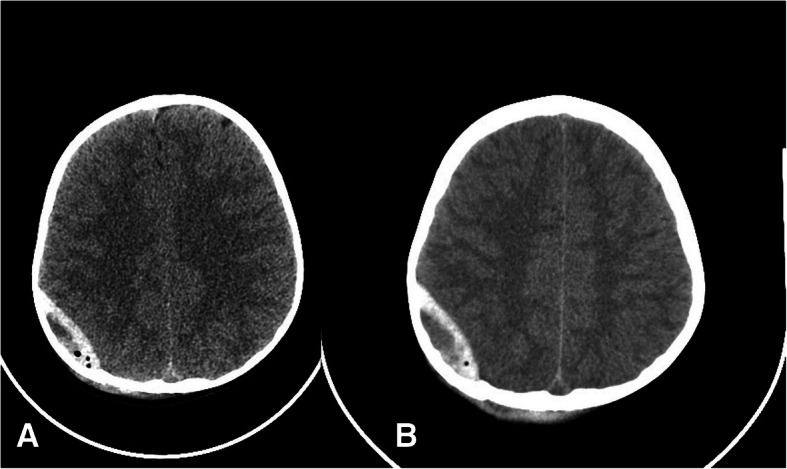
CT scan at admission (a) and 3 days after admission (b) of a child presenting a late clinical deterioration. The EDH was surgically evacuated
